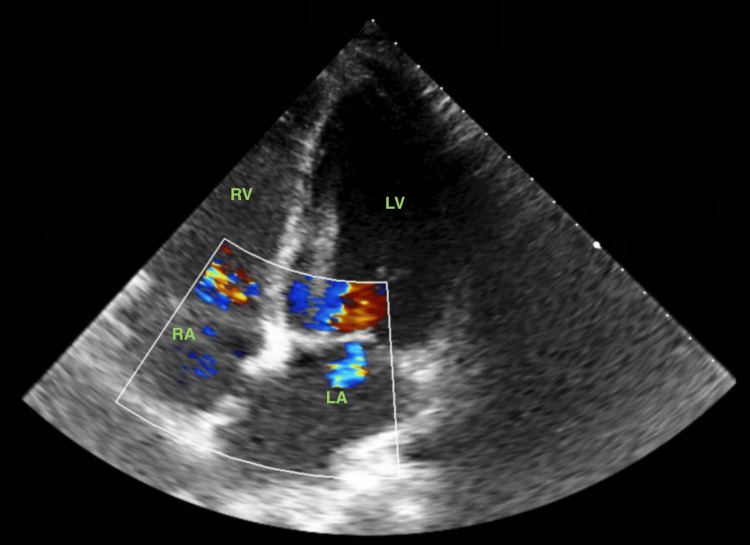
Doppler transthoracic echocardiography (4-chambers view) LA: left atrium; LV: left ventricle; RA: right atrium; RV: right ventricle This image was taken three months after surgical repair.
